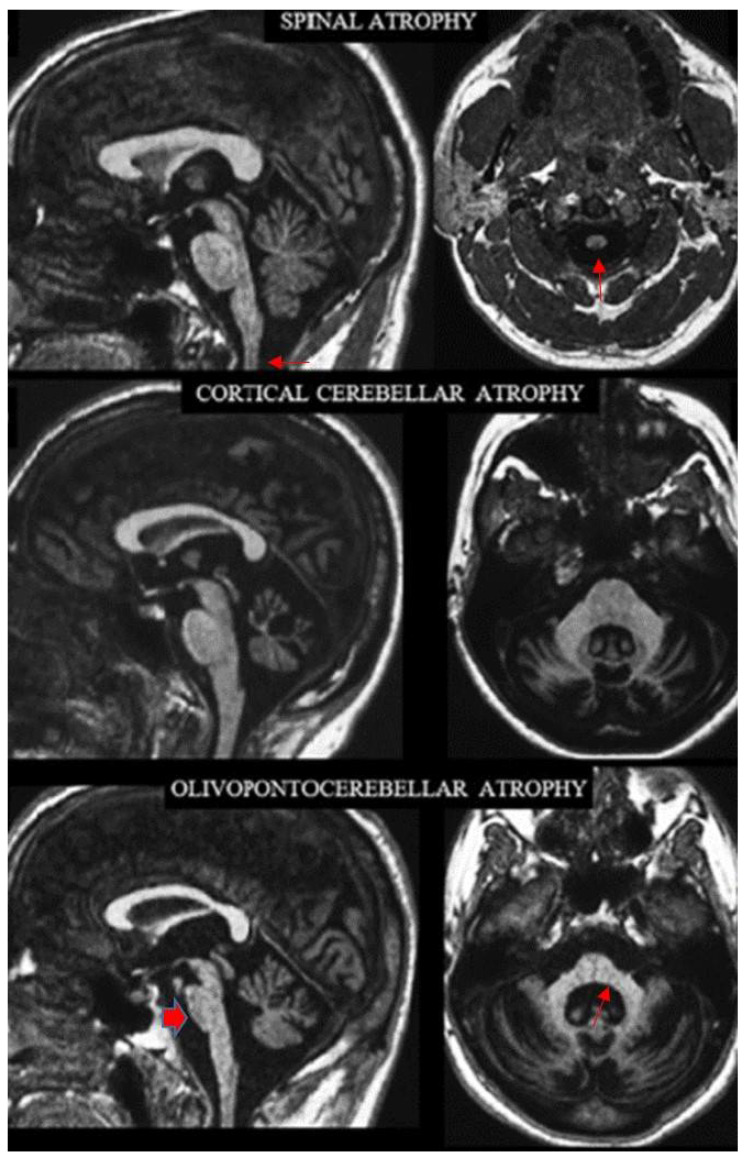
Sagittal (left column) and axial (right column) T_1_-weighted MR images in three exemplificative patients show the three CNS atrophy patterns underlying progressive ataxias (modified by ref. [29]). The typical features of spinal atrophy (SA), namely thinned medulla and cervical spinal cord (arrows) with normal volume of the pons and cerebellar vermis, are observed in a patient with Friedreich ataxia (top). Loss of bulk of the vermis and cerebellar hemispheres with enlarged interfolia spaces but normal volume of the pons, middle cerebellar peduncles and of the cervical spinal cord are the hallmark of cortical cerebellar atrophy (CCA) in a patient with sporadic adult-onset ataxia (SAOA) (mid). Atrophy of the brainstem, more pronounced in the inferior portion of the basis pontis (arrowhead), of the vermis, of the middle cerebellar peduncles (arrow) and of the cerebellar hemispheres characterizes olivopontocerebellar atrophy (OPCA) in a patient with SCA2 (bottom). Note in the bottom images the thinning of the superior cervical medulla that can be observed in SCA2 as result of secondary axonal and trans-synaptic degeneration.
